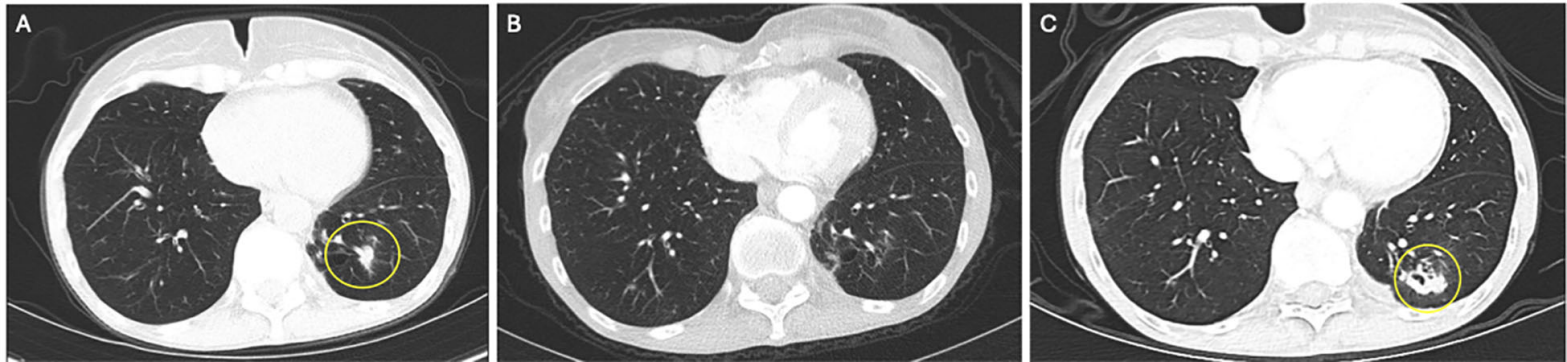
Radiographic imaging of lung metastases. (A) Left lower lobe metastasis at the site of a prior wedge resection before clinical trial enrollment. (B) R Resolution of the left lower lobe nodule two months after clinical trial enrollment. Stable 8mm right upper lobe nodule not pictured. (C) 25 months after trial enrollment, increased soft tissue and ground glass attenuation around the left lower lobe, presumed to be bronchiectatic changes and concerning for disease progression. A CT-guided biopsy was positive for metastasis.
